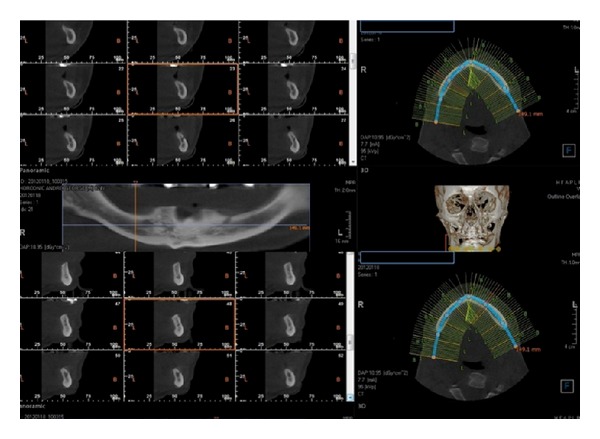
Preoperative CT dentascan imaging.
